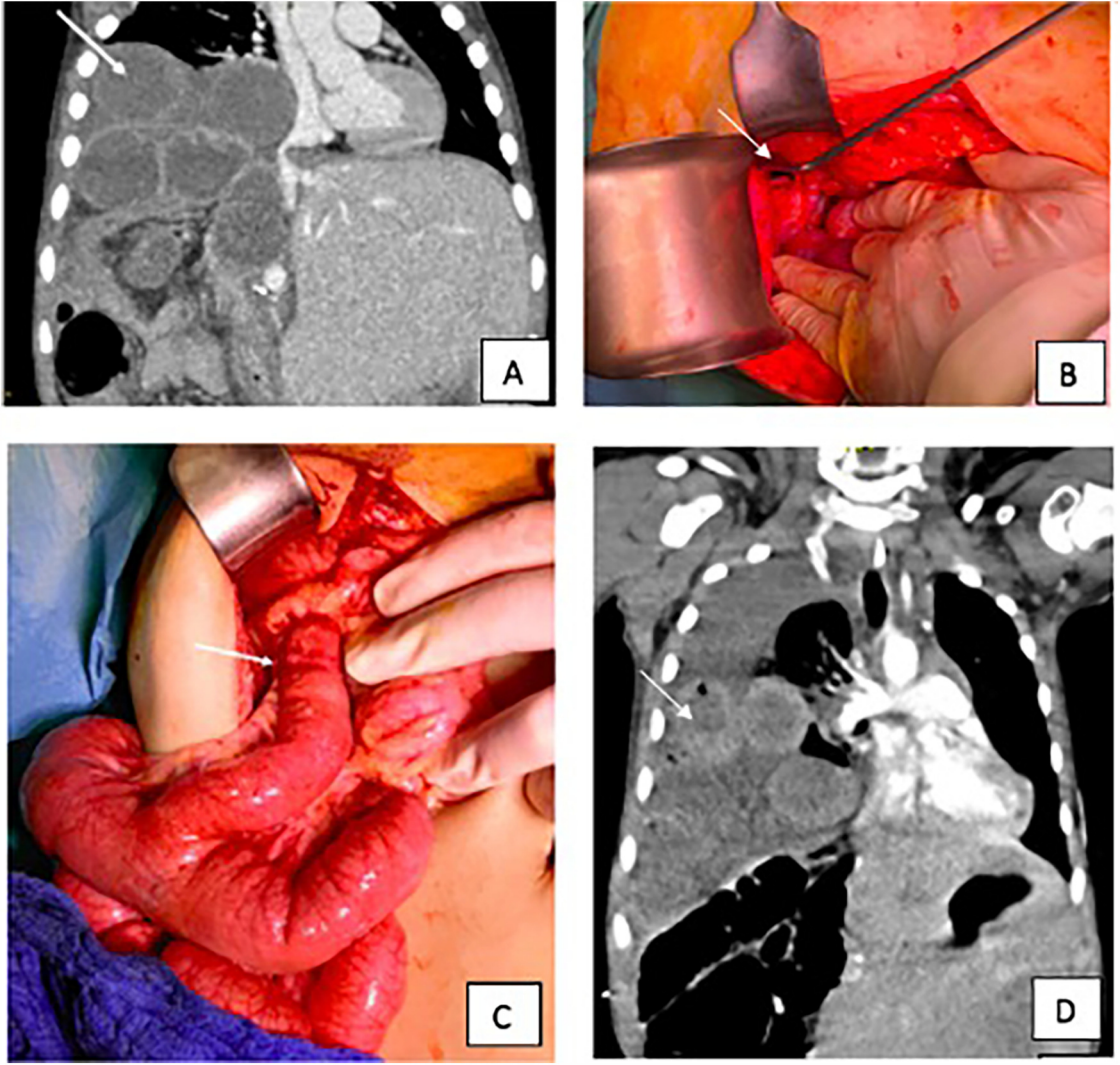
(A) Coronal view of CT image (with iodinated contrast); the arrows point at the herniated bowel loops (patient 1) (B) diaphragmatic defect (patient 1); the arrow points at the defect. (C) Herniated Roux-en-Y bilioenteric loop in the breached diaphragm (Patient 2); the arrow points at the herniated loop. (D) Coronal view of CT image (with iodinated contrast) at the time of first recurrence (Patient 3); the arrow points at the herniated bowel in the thoracic cavity.
